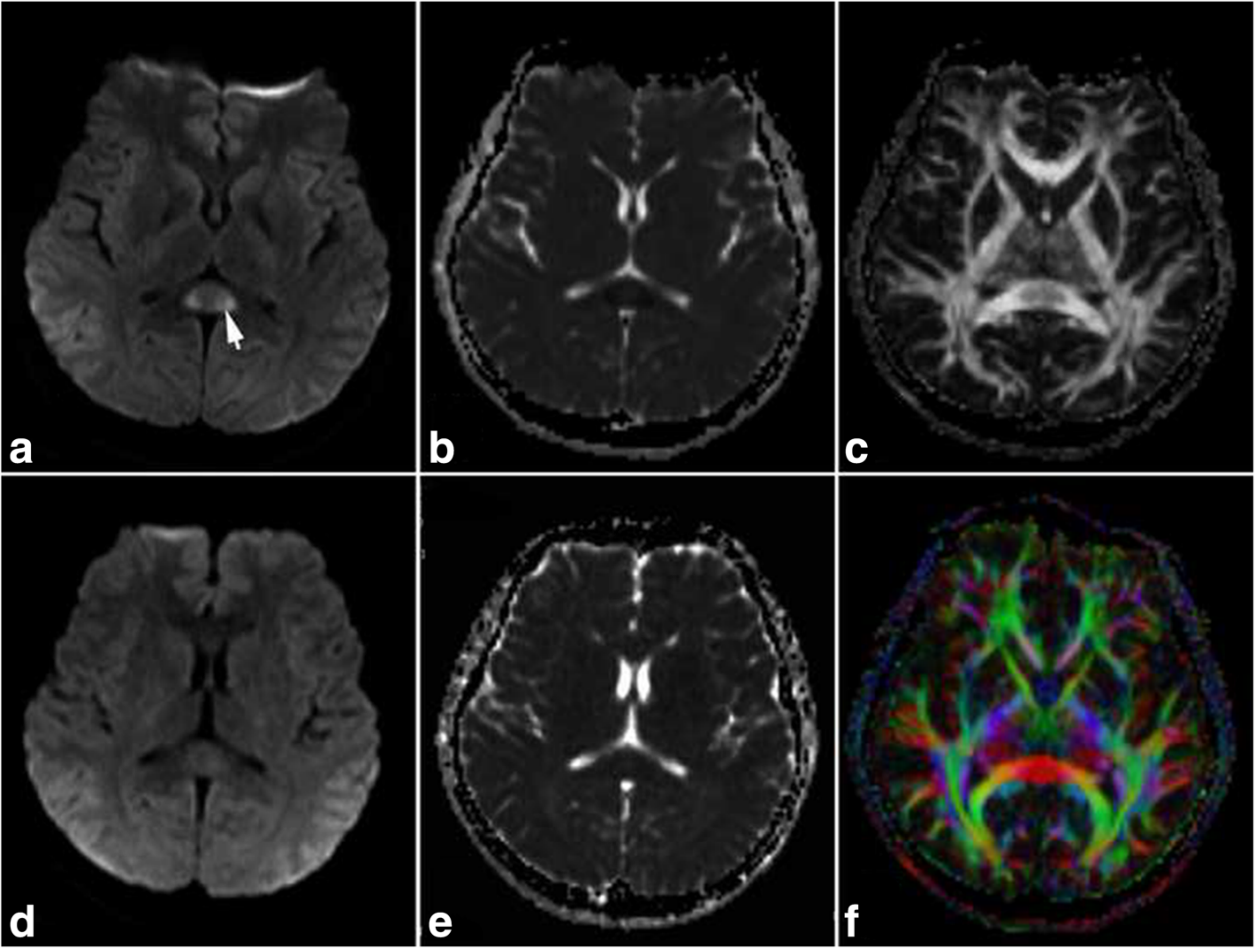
The cerebral MRI features of case 2. MRI revealed an isolated SCC lesion with obvious hyperintense on DWI (a, *arrow*), decreased ADC values (b), decreased fractional anisotropy value (c), and normally projecting direction of white matter fibers (f). At 16 days after initial MRI, second MRI showed the abnormalities of SCC nearly disappeared on DWI (d) and ADC (e)
